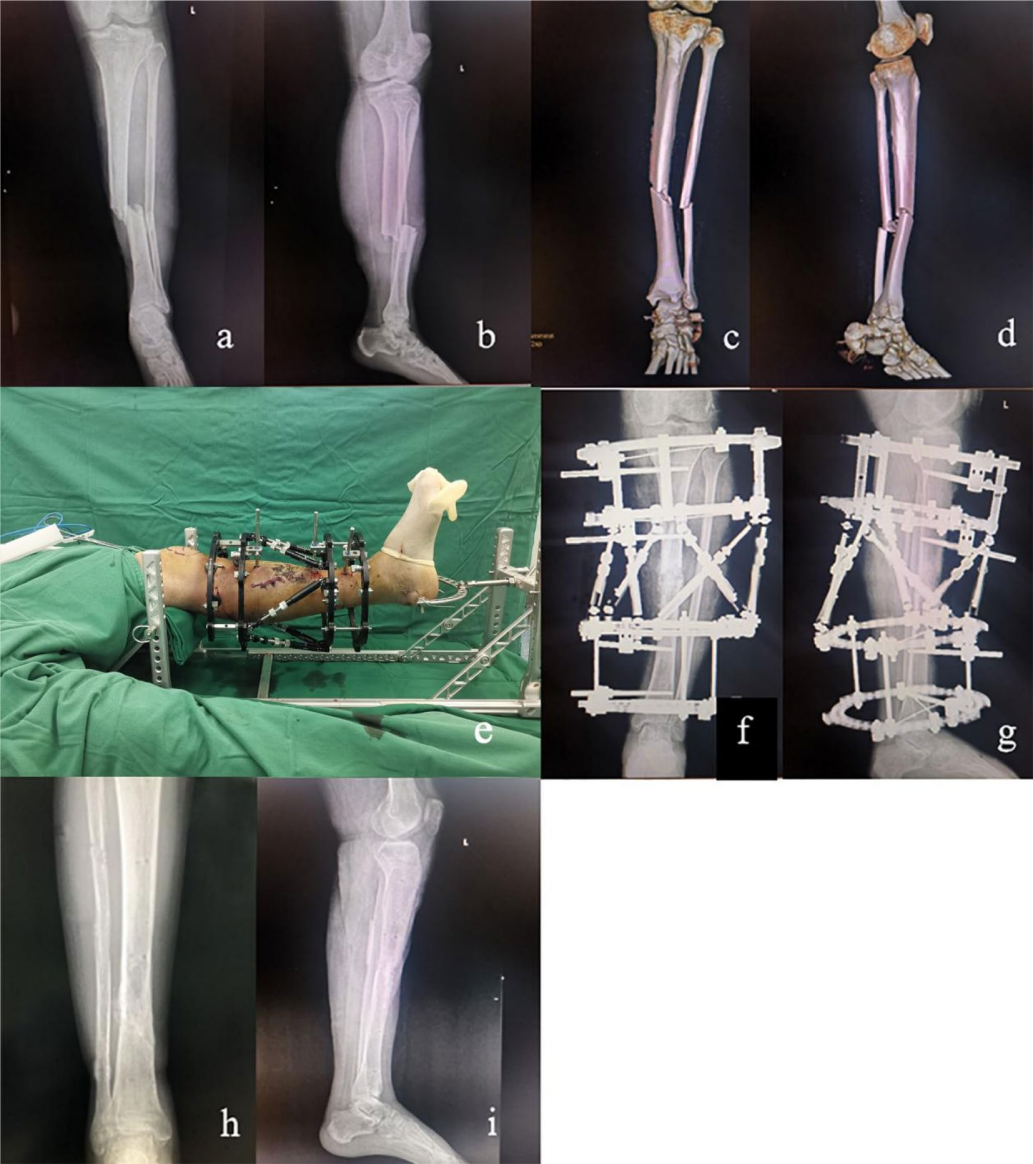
A 38‐year‐old male with Gustilo–Anderson classification IIIA open tibial shaft fracture (OTA/AO 42‐A2, simple oblique pattern) treated with double reverse traction‐assisted closed reduction and HEF. (a, b) Preoperative AP and lateral radiographs demonstrating a displaced oblique fracture with minimal comminution. (c, d) Preoperative 3D‐CT confirmed the simple oblique pattern without significant fragment separation. (e) Intraoperative photograph demonstrating the application of DRTA combined with HEF. (f, g) Immediate postoperative radiographs showing anatomical reduction with near‐perfect apposition of fracture fragments. (h, i) Final follow‐up radiographs at 6 months postoperatively (after HEF removal) showing solid bony union with excellent alignment.
